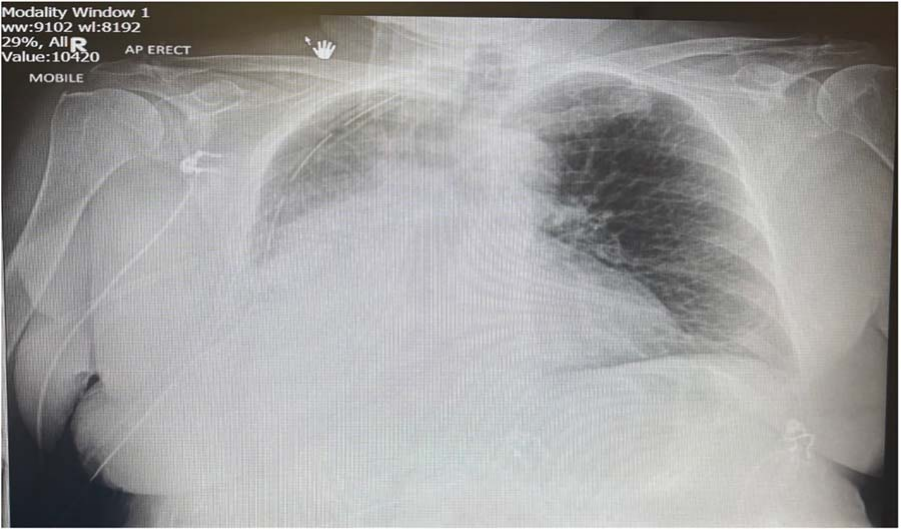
Portable chest X-ray Day 2 postoperatively. Chest X-ray depicts right middle and lower zone consolidation with associated pleural effusion.
